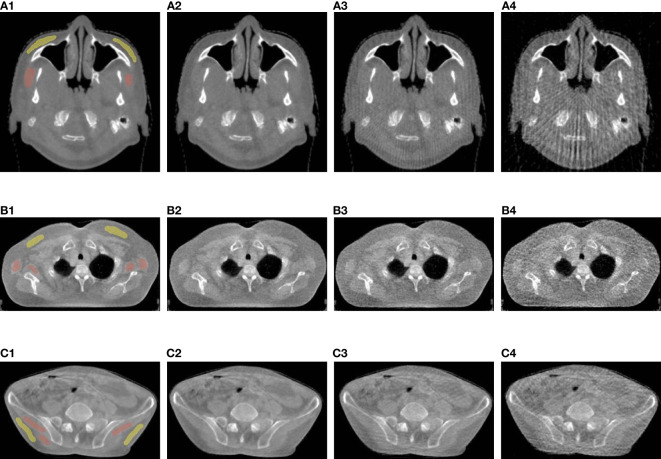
Visual image quality comparison of head (A-row), thorax (B-row), and pelvis (C-row) CBCT. The 1^st^ column shows full-dose images, and 2^nd^, 3^rd^ and 4^th^ columns show *S_1/2_
